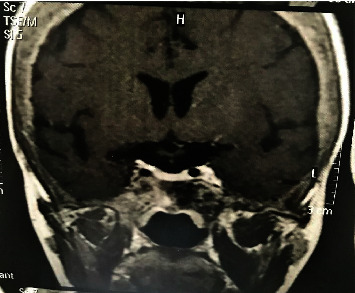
MRI in T1 showing significant regression of pituitary fossa lesion one year after parathyroidectomy.
